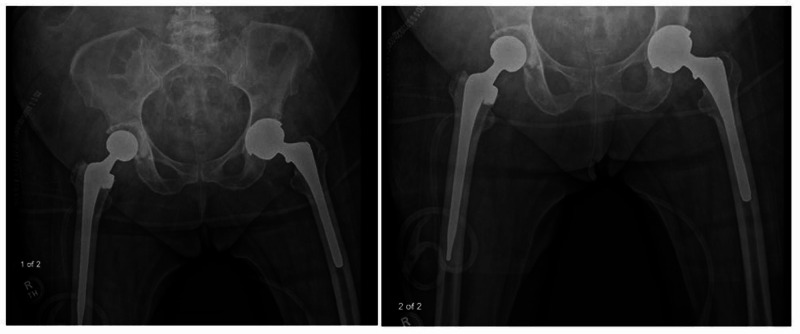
Final postoperative plain radiographs demonstrating this alternative one-stage exchange arthroplasty technique on the right hip.
